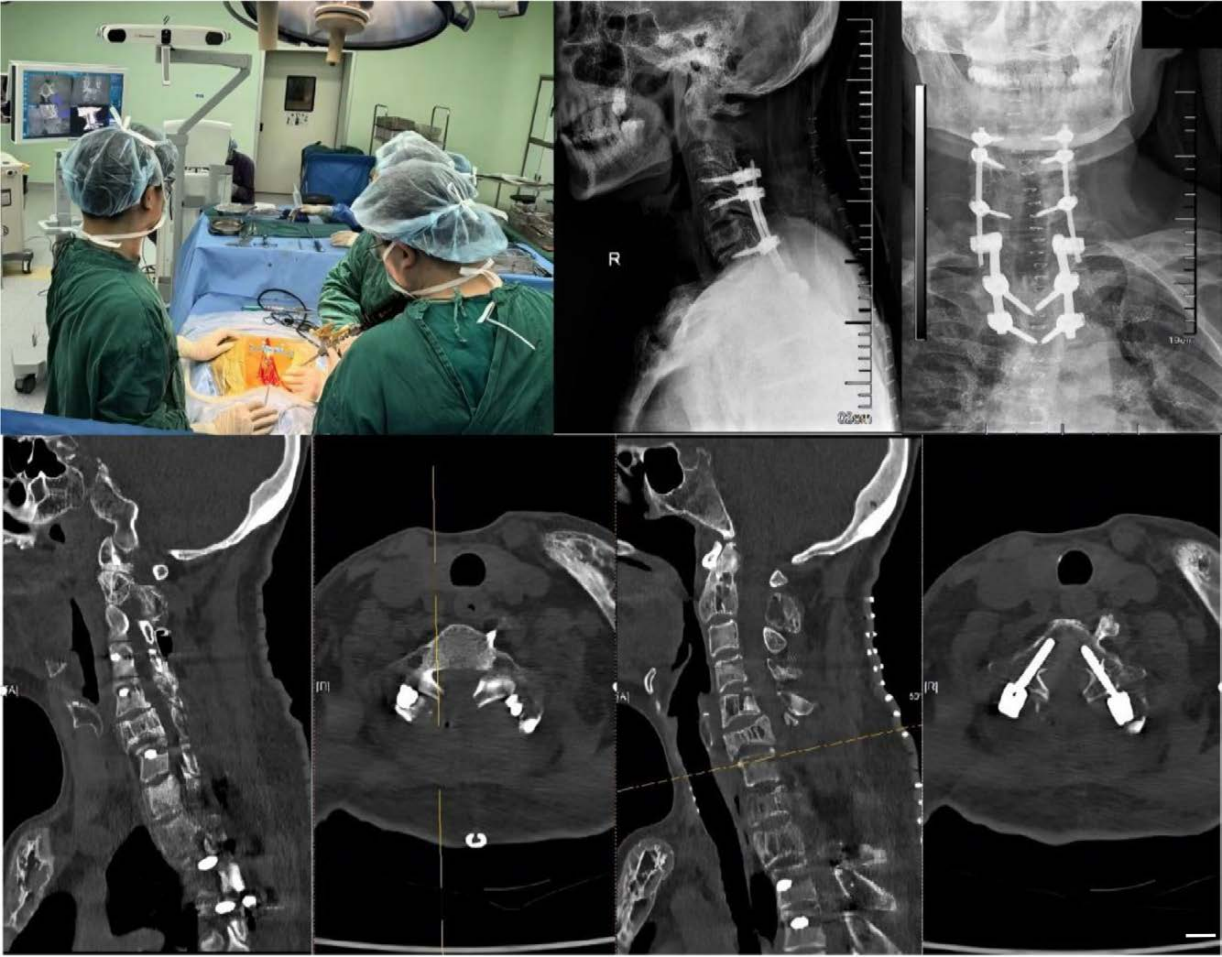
Postoperative imaging after internal fixation and decompression. X-ray and CT images confirm successful implantation of the internal fixation, which enhanced cervical stability and markedly reduced spinal canal stenosis and cord compression (scale bar = 2 cm). CT = computed tomography.
